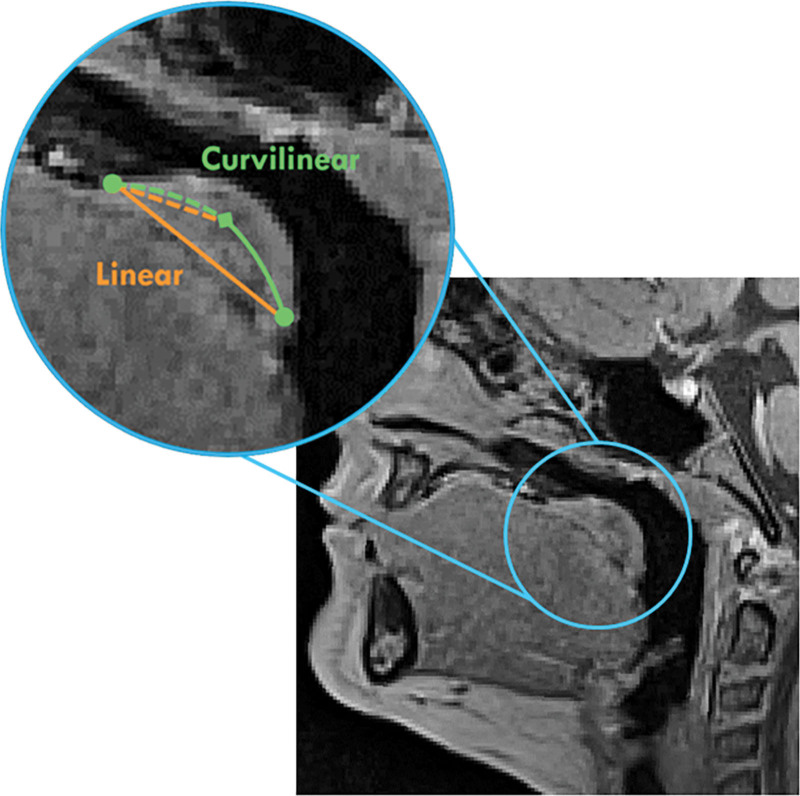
Comparison between linear (orange) and curvilinear (green) measures of velar length (solid lines) and effective velar length (dashed lines) on midsagittal MRI. The superior circular dot (green) indicates the location of the PMP, which is used as the starting point for measurements. The uvular tip is noted by the inferior circular dot, which notates the final landmark for measurements. The midline of the levator veli palatini muscle, as viewed on a midsagittal slice, is indicated by the diamond (green). This landmark can be used to obtain measurements of effective velar length as well as to track the course of the curvilinear velar length measurement.
